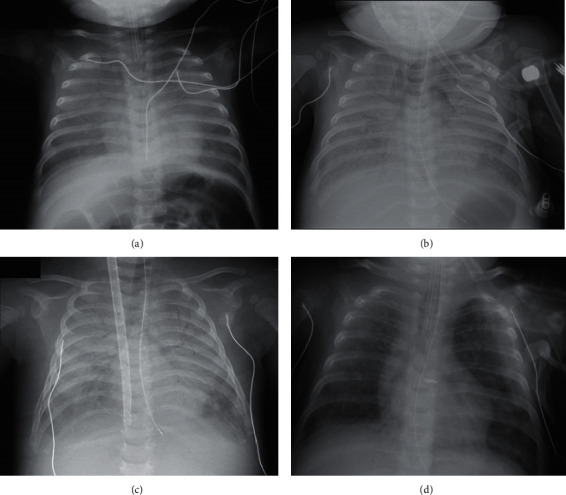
Chest X-rays taken on admission (a): on the day before cannulation and VV-ECMO commencement (b), on the day of VV-ECMO commencement (c), and on the day before extubation (d). Note the Avalon Cannula projecting in front of the superior and inferior vena cava in (c).
